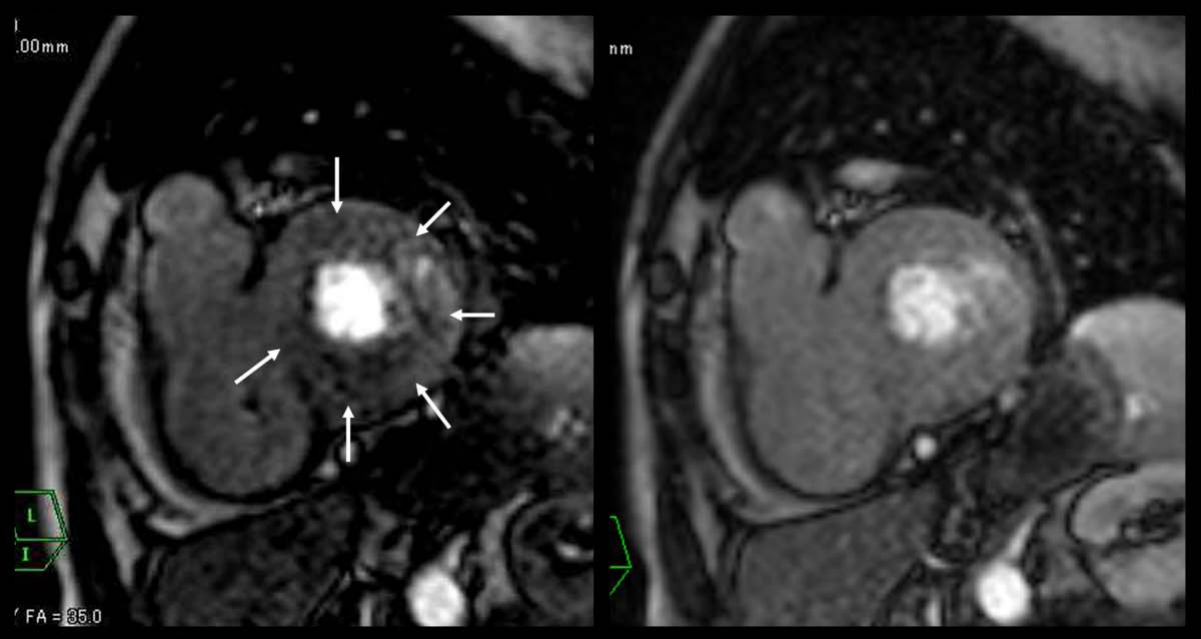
Early perfusion defect of microvascular impairment without myocardial fibrosis or myocarditis. Perfusion MRI under stress (left figure) showed non-segmental circumferential perfusion defect (arrows). Perfusion MRI at rest (right figure) showed no defects. In this case, delayed enhanced images showed no enhancement (not shown).
